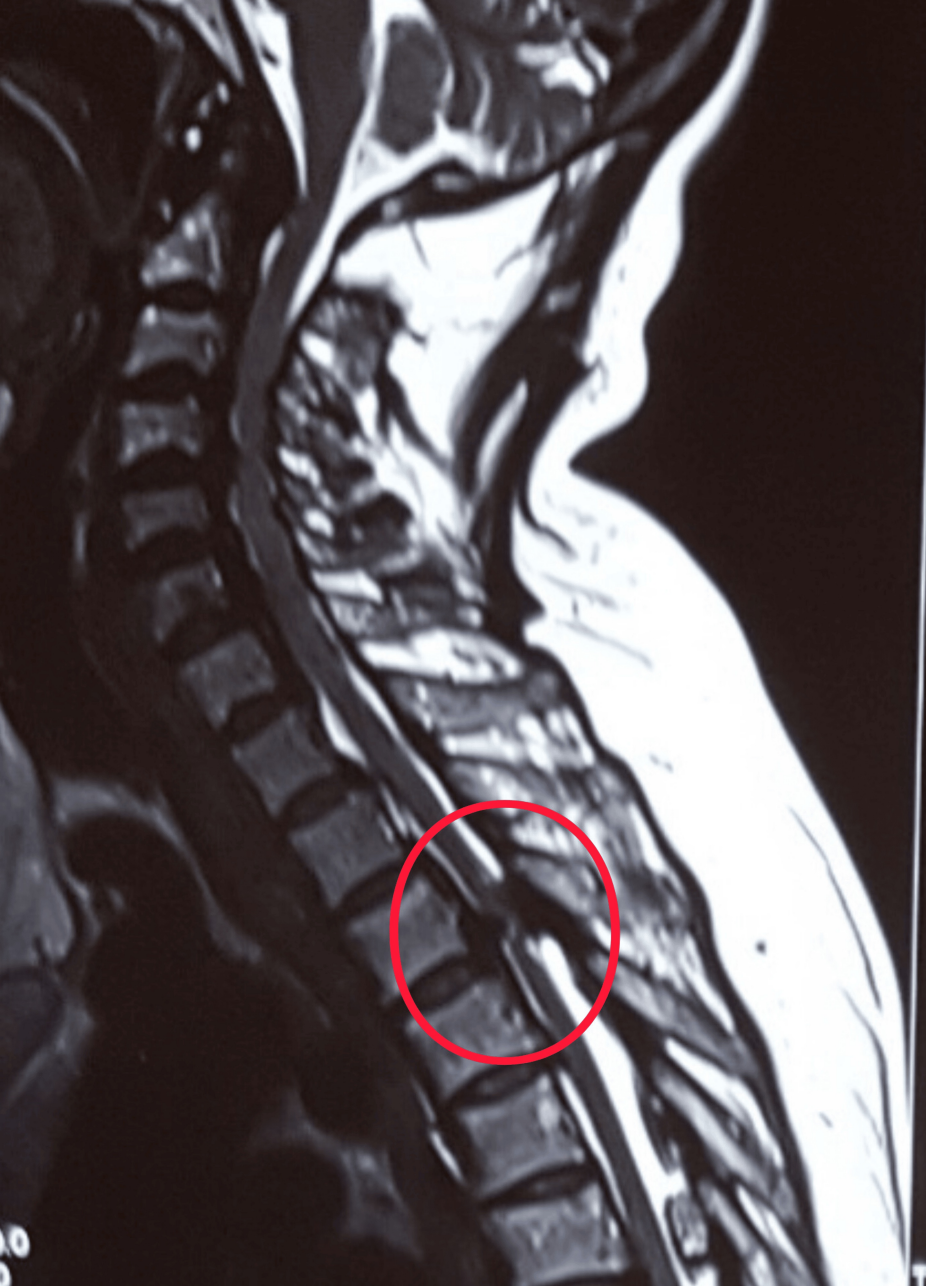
Pre-operative magnetic resonance imaging (MRI) demonstrates severe spinal cord compression at the T3-T4 level with associated T2 signal change (red circle). T3 denotes thoracic vertebral segment 3, and T4 denotes thoracic vertebral segment 4.
